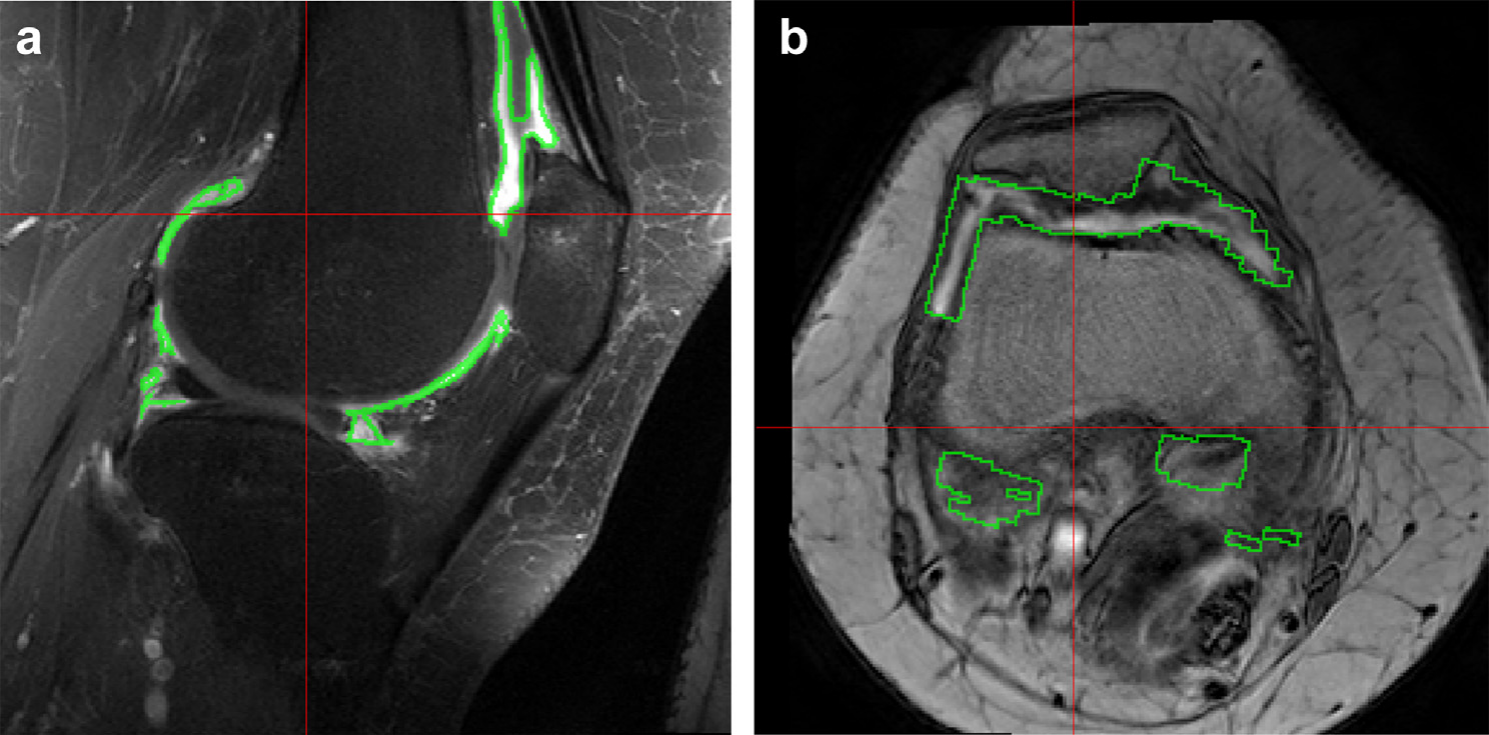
(a) Sagittal post contrast T1-weighted fat suppressed turbo spin-echo image after intravenous contrast enhancement with the synovial segmentation ROI outlined in green. Manual segmentation of the synovial tissue was performed on the sagittal post-contrast T1W FS image by a single observer. Segmentations were carried out paired, blinded to order. Using computer image analysis, cartilage was excluded by thresholding using the sagittal pre-contrast scan and the synovial fluid using the sagittal post-contrast scan (see also Ref. 4) The synovial volume is shown in Table II. (b) axial image from the DCE MRI sequence showing the placement of the ROI outlined in green. The ROI was determined from the pre and post contrast sagittal images and was then transferred to the axial dynamic dataset (truncated by the field-of-view of the dynamic images) for the DCE-MRI analysis.
